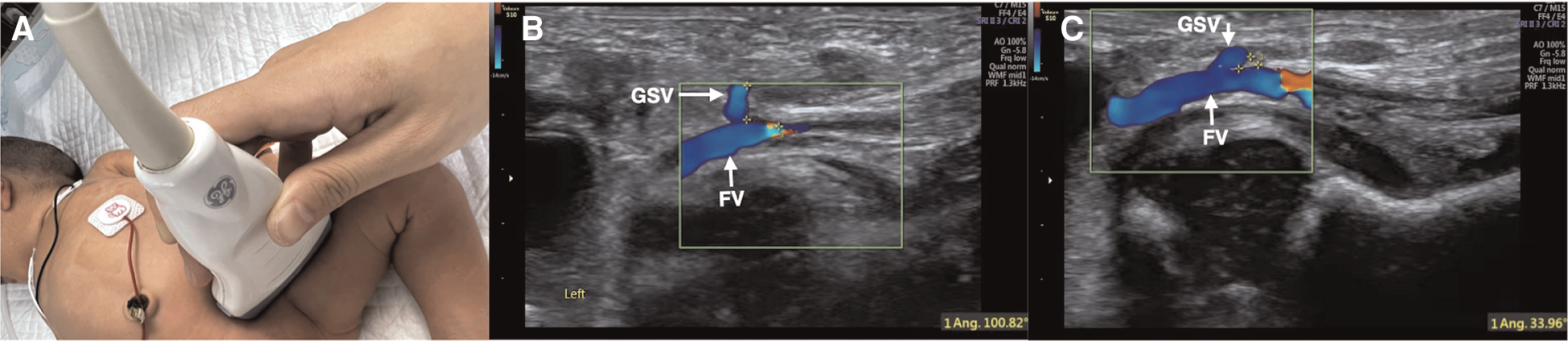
Color Doppler ultrasound measuring the angle between GSV and FV. Color Doppler ultrasound was used to assess the course conditions of bilateral GSV. The probe is placed in the groin (A). Measuring the angle between GSV and FV (B: angle = 100°; C: angle = 33.9°). The GSV on one side with a smaller angle between the two veins was selected for catheterization (C).
